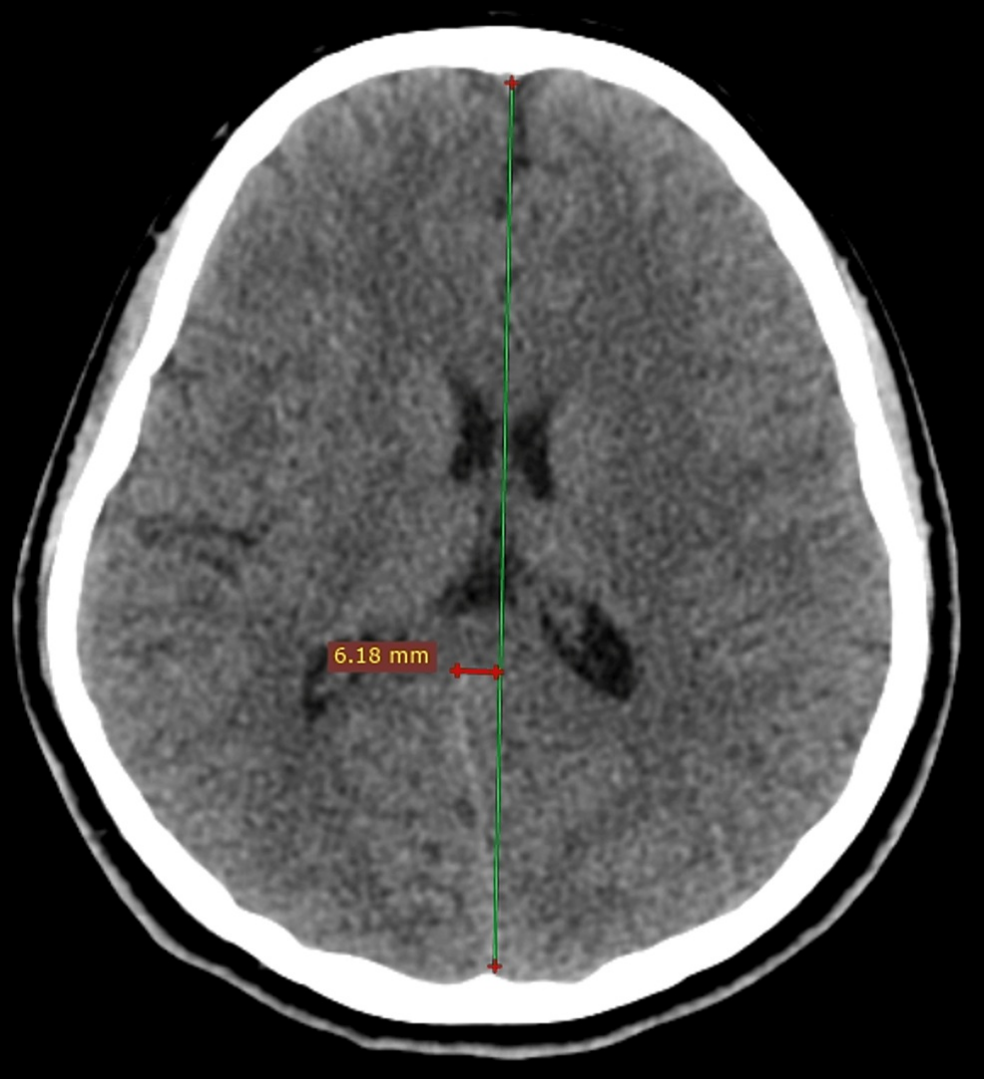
CT, brain window, axial image. The slight rightward shift of posterior falx due to herniation of the occipital lobe through the defect. CT: computed tomography
